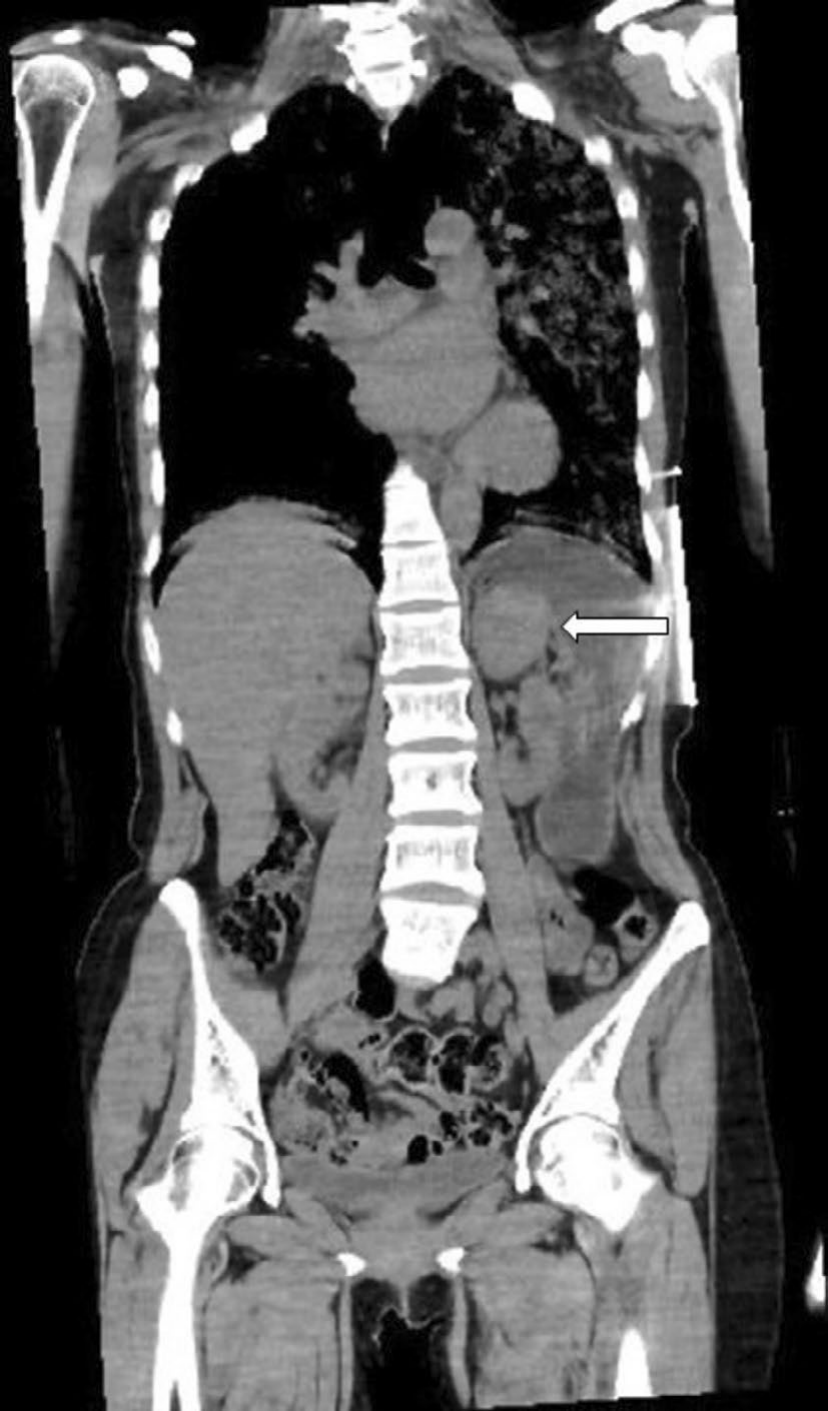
Abdominal computed tomography revealed a smooth, round tumor measuring 45 mm in diameter.
